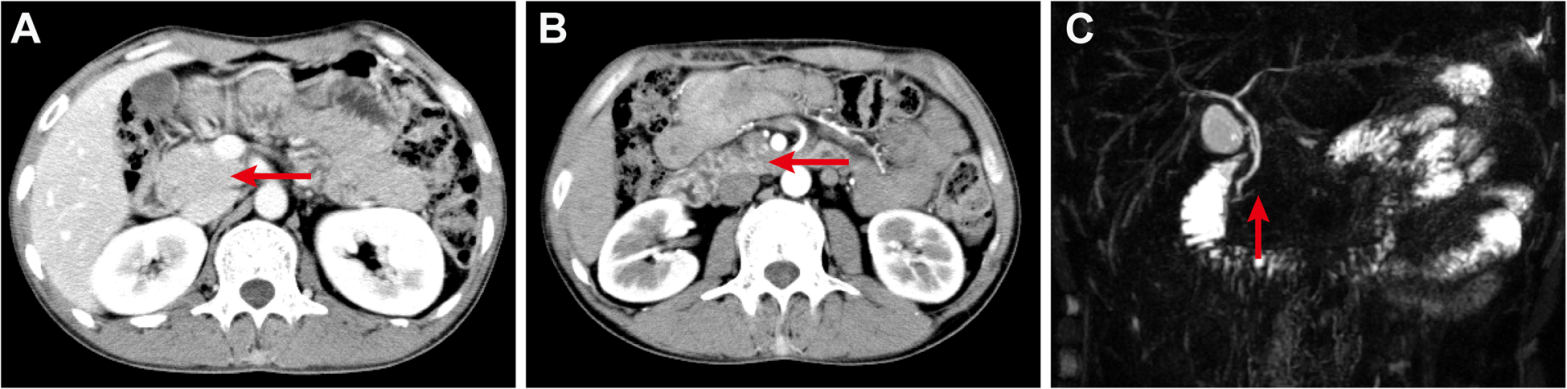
Contrast abdominal computed tomography scan showed the pancreatic head (a, red arrow), whereas the pancreatic body and tail are absent (b, red arrow). Magnetic resonance cholangiopancreatography demonstrated the absence of the dorsal pancreatic duct (c, red arrow)
